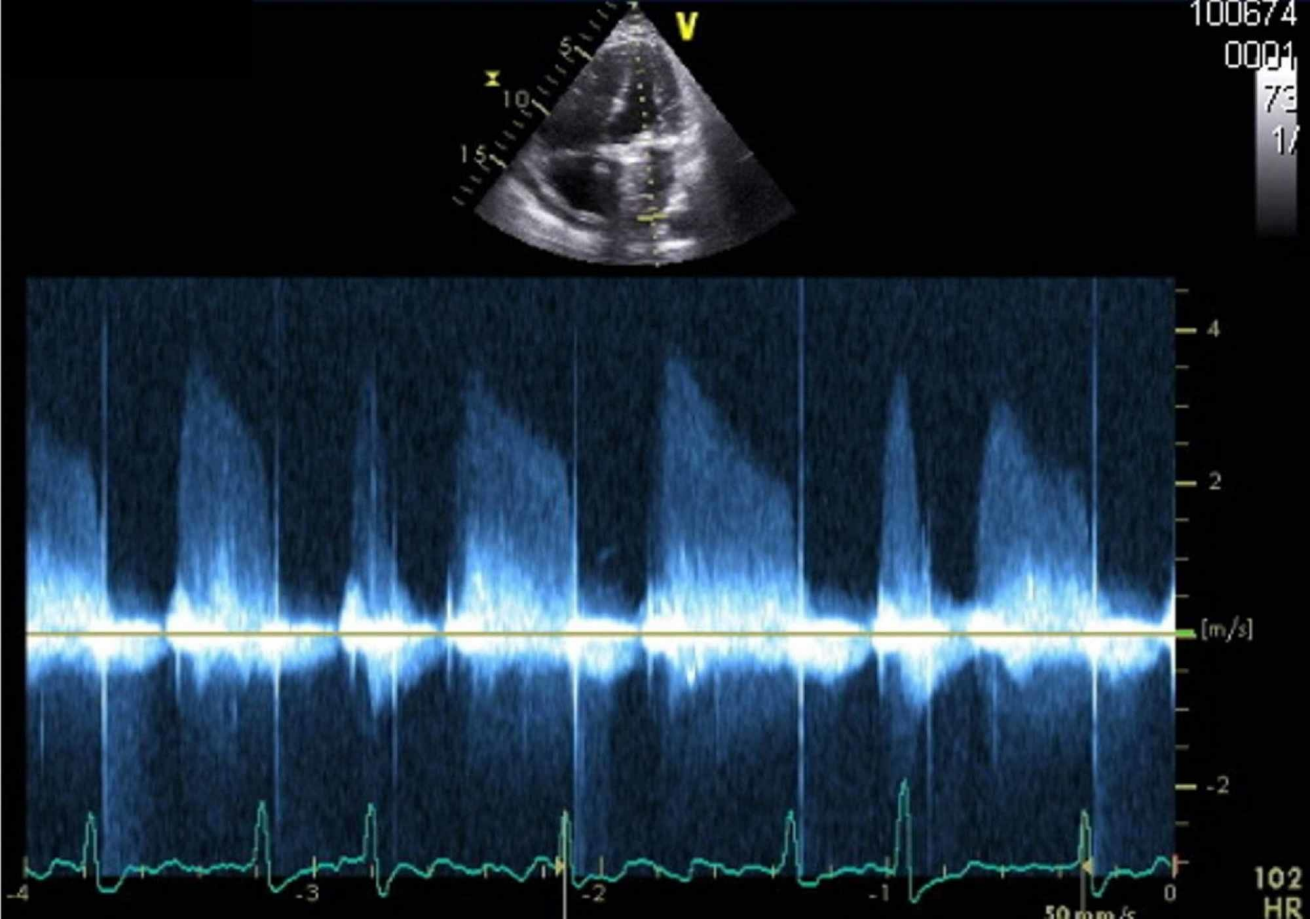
Transthoracic echocardiography, apical four chamber view. Continuous doppler across the mitral valve, demonstrating elevated trans-mitral gradient of 23 mmHg (averaged over five beats) at a heart rate of 102 beats/min.
